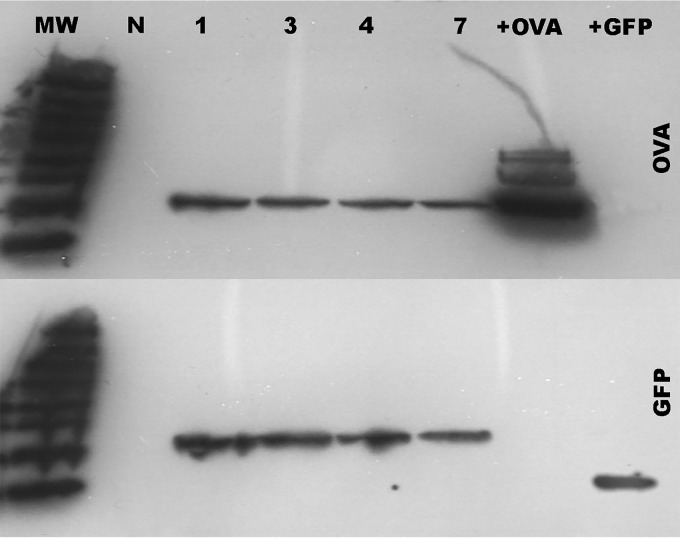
Western analyses of transposon-transformed Brucella lysates. Equivalent amounts of Brucella clones were lysed, denatured in SDS-Laemmli sample buffer, PAGE separated, and blotted. Antibodies to chicken ovalbumin (OVA) or green fluorescent protein (GFP) were used along with horseradish peroxidase (HRP) secondary antibody. Chemiluminescence was detected by X-ray film. Lanes: N, parental Brucella; 1, 3, 4, and 7, transposon-transformed Brucella clones; +OVA, chicken ovalbumin; +GFP, green fluorescent protein; MW, molecular weight marker.
